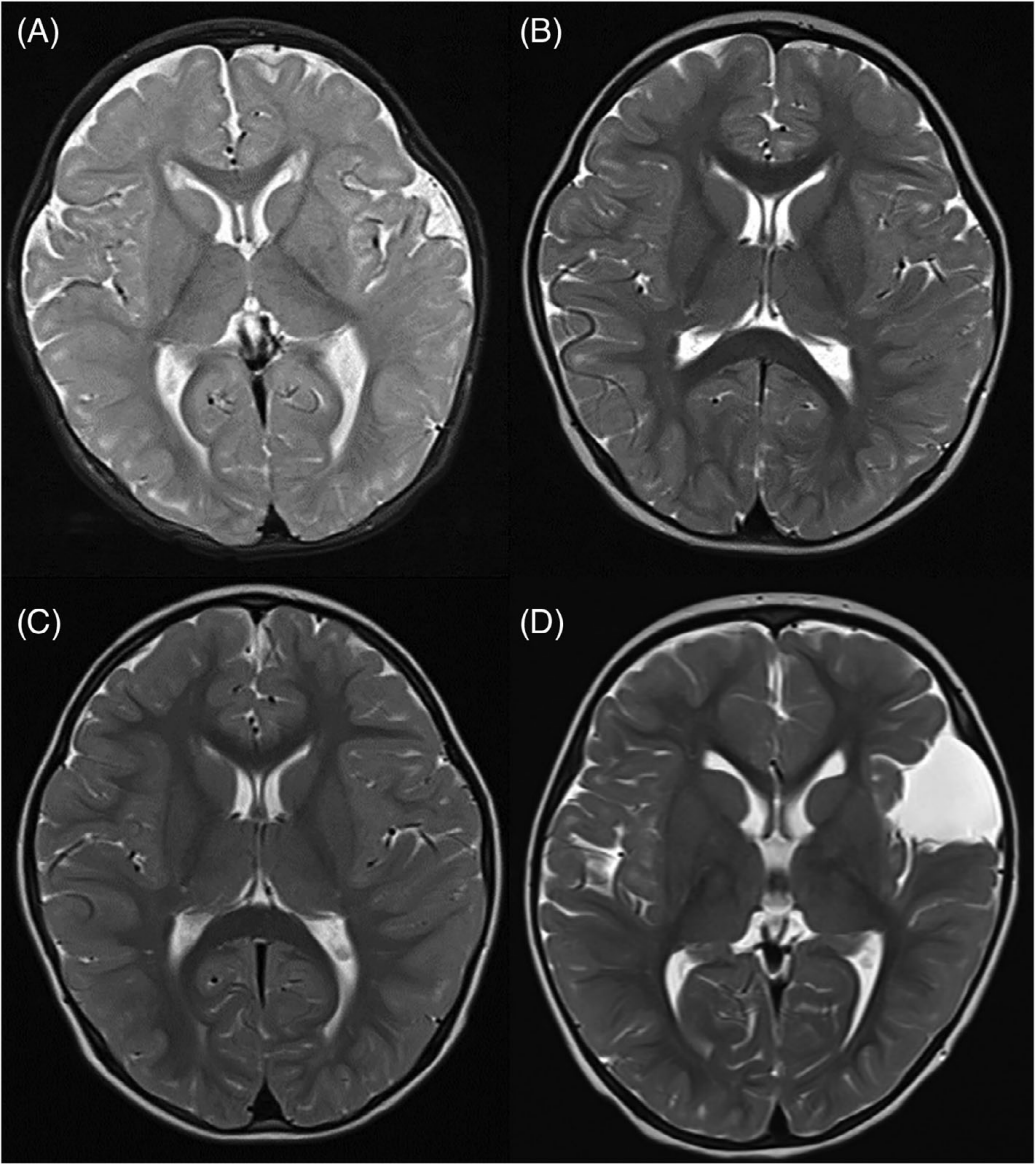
MRI brain (T2‐weighted images) in P1; (A) at 12 months of age showing delayed myelination in cerebral hemispheres, (B) at 3 years of age showing some ‘catch‐up’ of myelination, with incomplete myelination in several gyri, and (C) at 4.75 years of age, 18 months after commencing triheptanoin, showing almost age‐appropriate myelination. (D) MRI brain (T2‐weighted images) in P2 at 3.2 years of age, showing incompletely myelinated cerebral gyri, mild global volume loss and a left frontotemporal arachnoid cyst.
